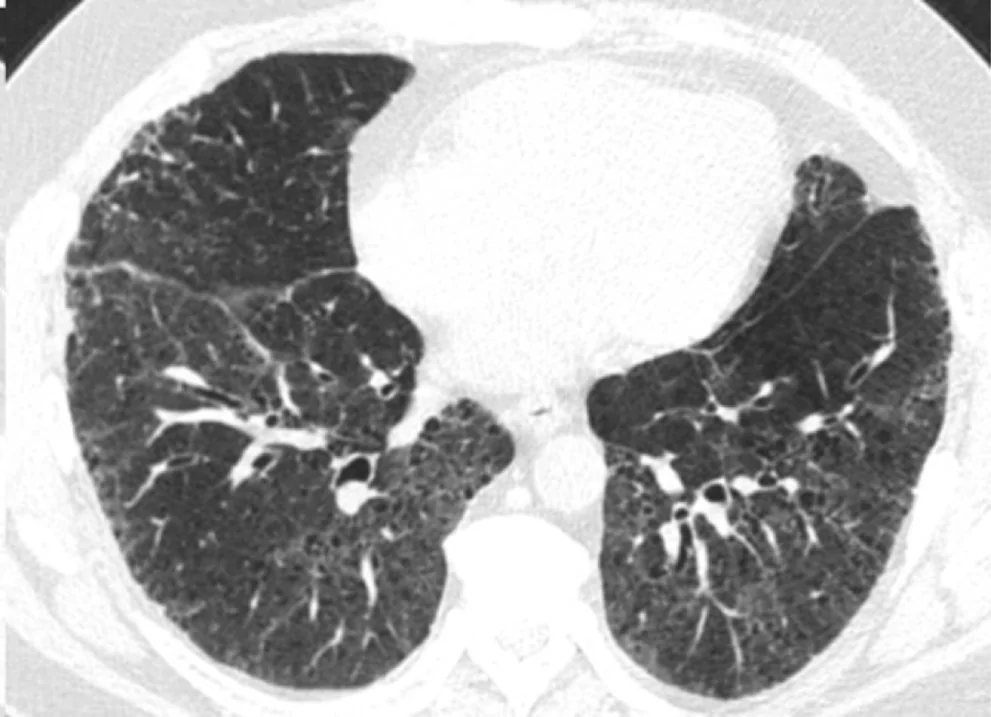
High‐resolution chest CT (lung window) demonstrating fibrotic interstitial lung disease of indeterminate pattern, characterised by bilateral mild ground‐glass opacities and reticular abnormalities associated with traction bronchiectasis.
